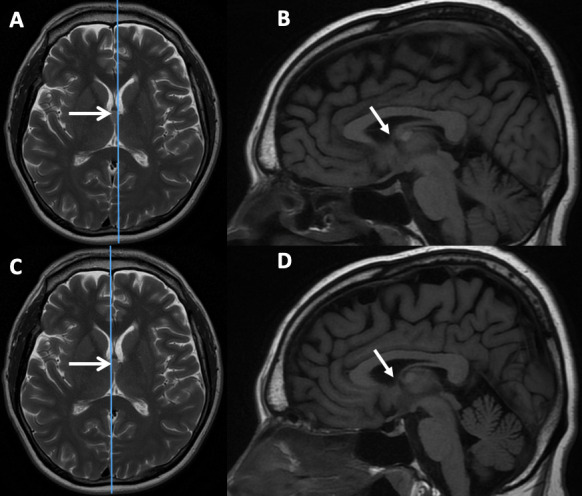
Axial T2-weighted and sagittal T1-weighted MRI sequences showing a colloid cyst (open arrows) arising from the middle part of the third ventricle. The parasagittal cuts to the right (B) and left (D) of midline show that the foramen of Monro is not obstructed (closed arrows) by the colloid cyst, despite the appearance on the T2 axial, therefore the risk of developing symptomatic hydrocephalus requiring treatment is low. The patient is asymptomatic and has been followed for 4 years with no change in MR appearance or clinical features.
